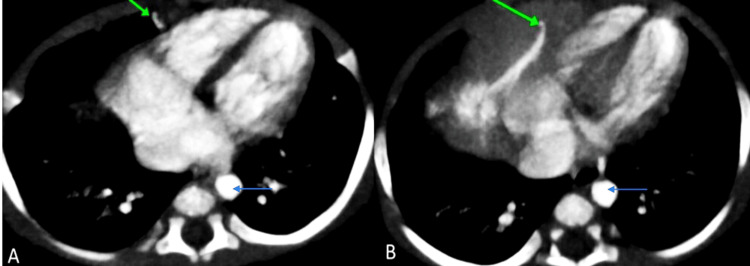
Axial section of contrast-enhanced CT images showing (A) lesion in segment VIII (green arrow) and intense enhancement of IVC (blue arrow) suggestive of right ventricular strain, (B) supplied by right internal mammary artery (green arrow) - likely musculophrenic branch CT: computed tomography; IVC: inferior vena cava
